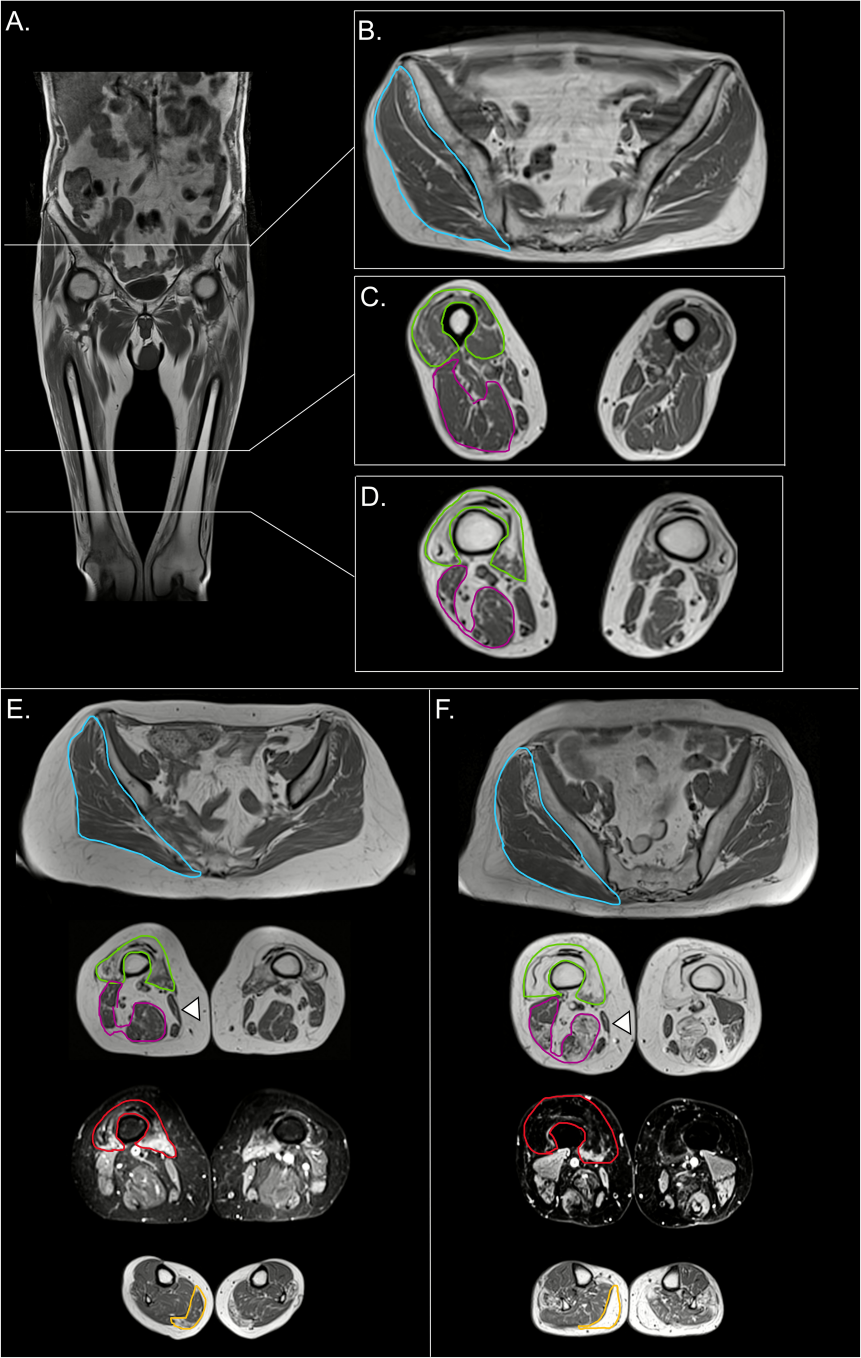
Refined MRI criteria.
